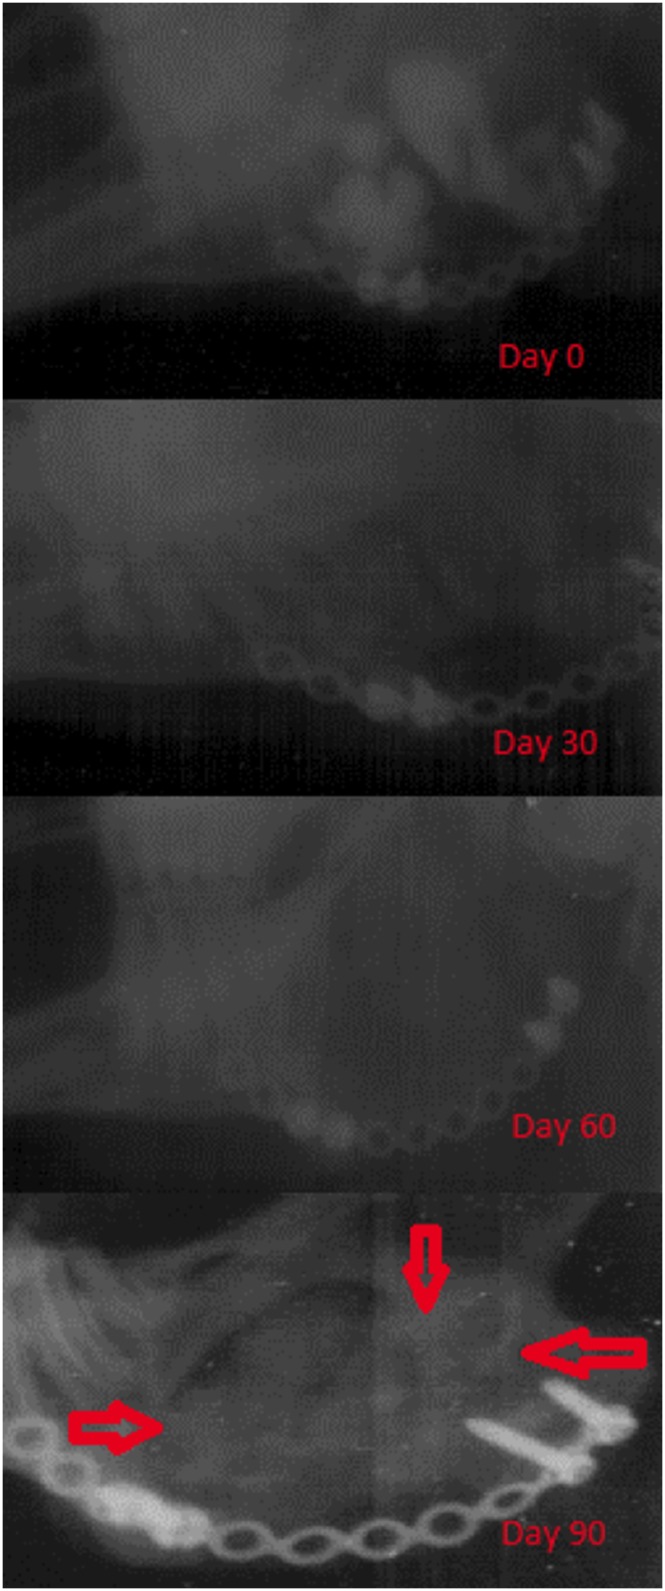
Radiographic images taken at different time points. A) immediately after surgery which shows the injected cement as radio- opaque patches, B) One month after surgery showing the disappearance of radio-opaque patches of cement, C) Two months following surgery, the radiograph shows homogeneous radio-opaque shadow, D) Three months postoperative radiograph showing radio-opaque mass occupying the area of the created defect.
